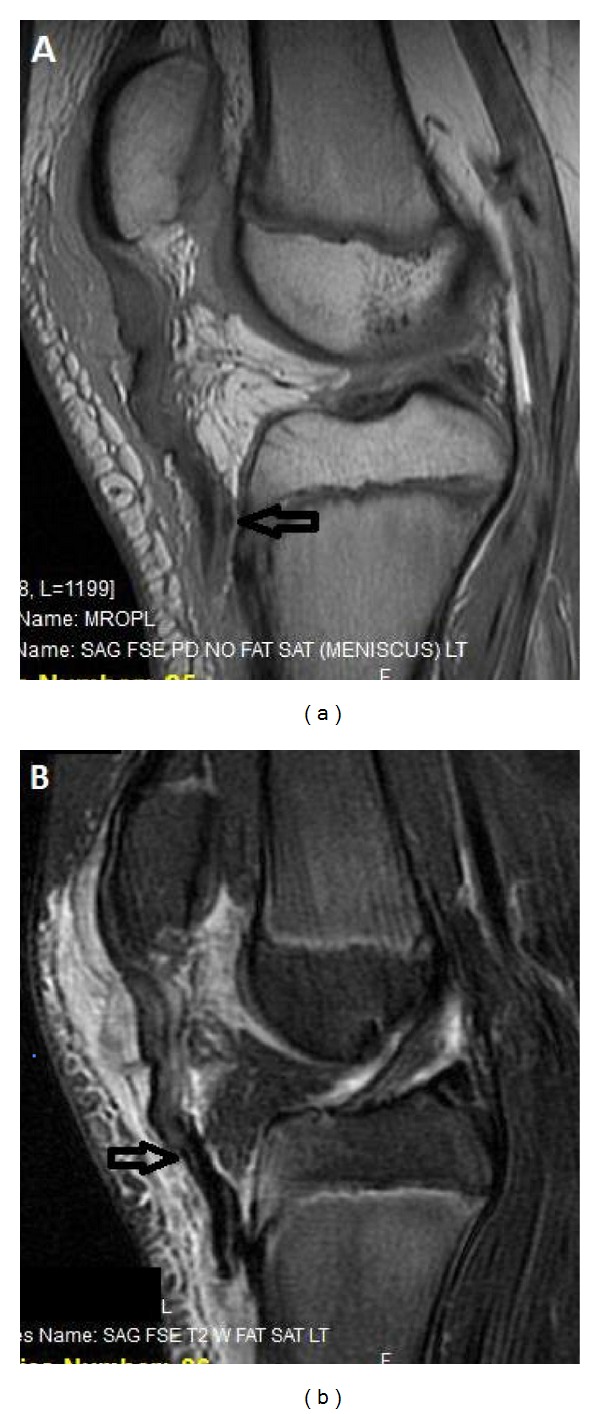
(a) Left knee MRI shows patellar tendon avulsion with the arrow pointing to the small bone fragment. (b) MRI demonstrates patellar tendon avulsion from its distal attachment on the right side. The arrow marks the distal extent of patellar tendon with the periosteal flap attached distal to it.
